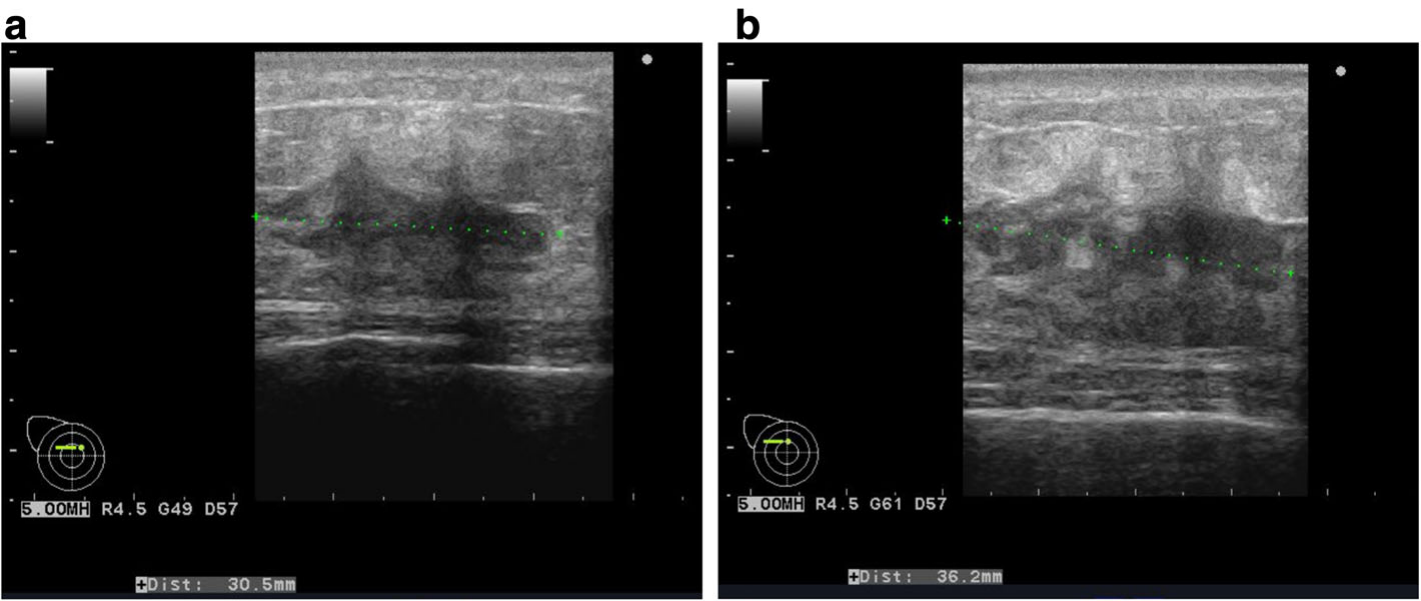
a Ultrasonographic images of the patient with the tumor. The inside of the tumor had a low echoic area, and the tumor borders were unclear. b The tumor grew larger 2 months later
